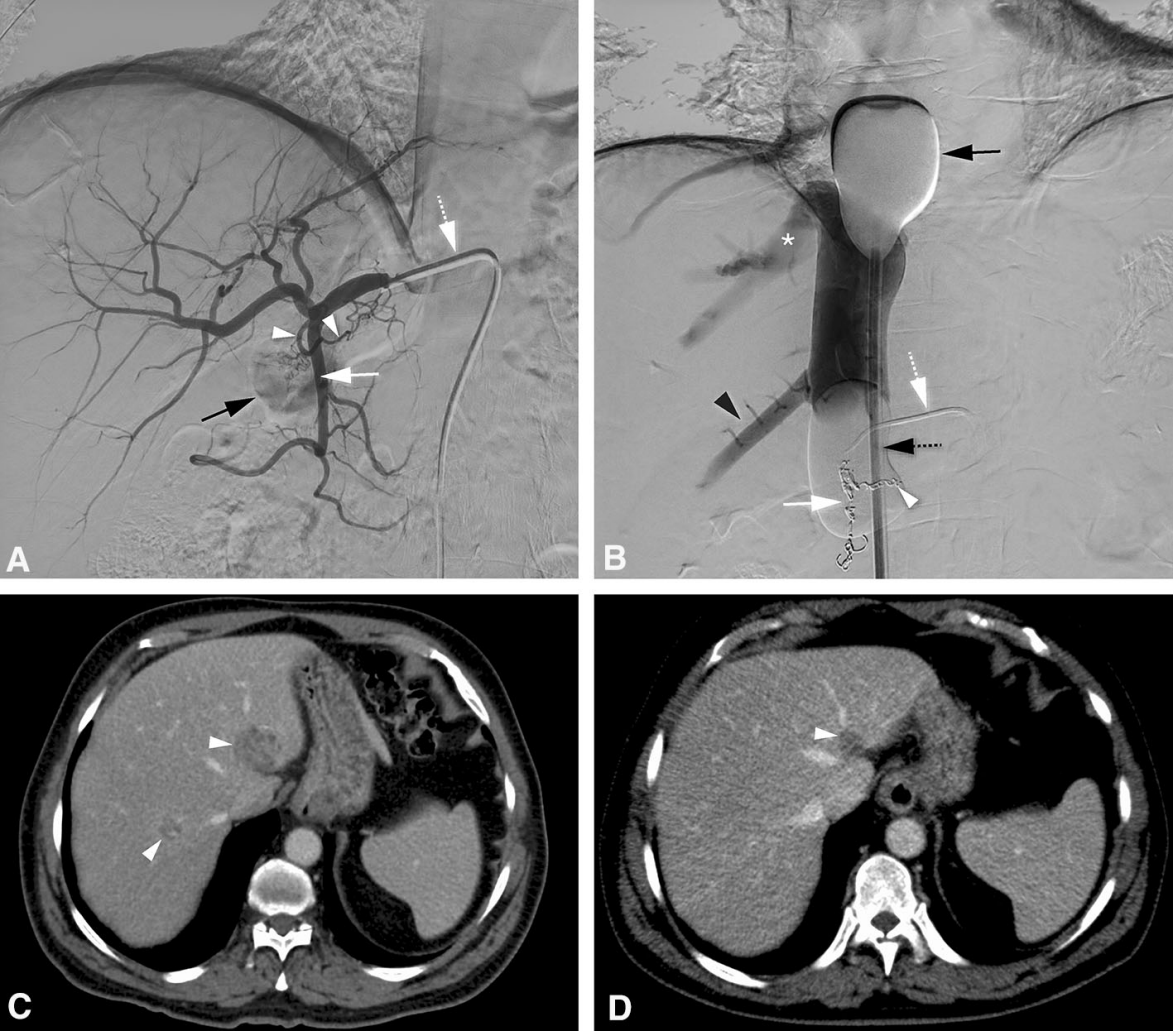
Percutaneous hepatic perfusion with melphalan (M-PHP) in a 66-year-old man with bilobar liver metastases from ocular melanoma. (a) Pretreatment angiographic image from the common hepatic artery (CHA) shows a right gastric artery (RGA, white arrowheads) and gastroduodenal artery (GDA, white arrow). Also a 5F macrocatheter in the CHA (dotted white arrow) and the duodenal bulb (black arrow) are shown. The RGA and GDA were successfully coiled. (b) Postero-anterior image during venography. The cranial balloon (black arrow) is inflated at the atriocaval junction to prevent flow to the right atrium, and the caudal balloon (dotted black arrow) is inflated in the infrahepatic inferior vena cava (IVC) to prevent retrograde flow to the infrarenal IVC. A 2.7F microcatheter was inserted through the macrocatheter (dotted white arrow) and placed into the proper hepatic artery for the infusion of melphalan. The right hepatic vein (asterisk) and accessory right inferior hepatic vein (black arrowhead) are opacified. Note the coils in the RGA (white arrowhead) and GDA (white arrow). (c) Axial CT image in portovenous phase before treatment shows a metastasis in liver segment II and VII/VIII (white arrowheads). A third lesion in segment VI is not shown. (d) Axial CT image in portovenous phase after two M-PHP procedures shows reduction in size of the metastasis in liver segment II (white arrowhead). The other two lesions showed a complete radiological response
